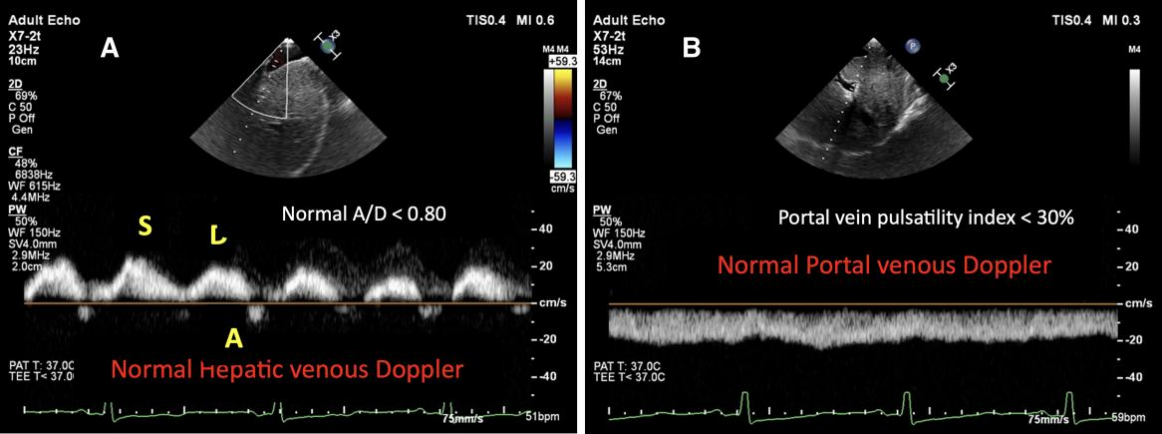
Normal hepatic venous Doppler waveform shows S, D, and a waves (panel *A*) (TEE image); normal portal venous Doppler shows continuous waveform with no pulsatility (panel *B*) (TEE image).
